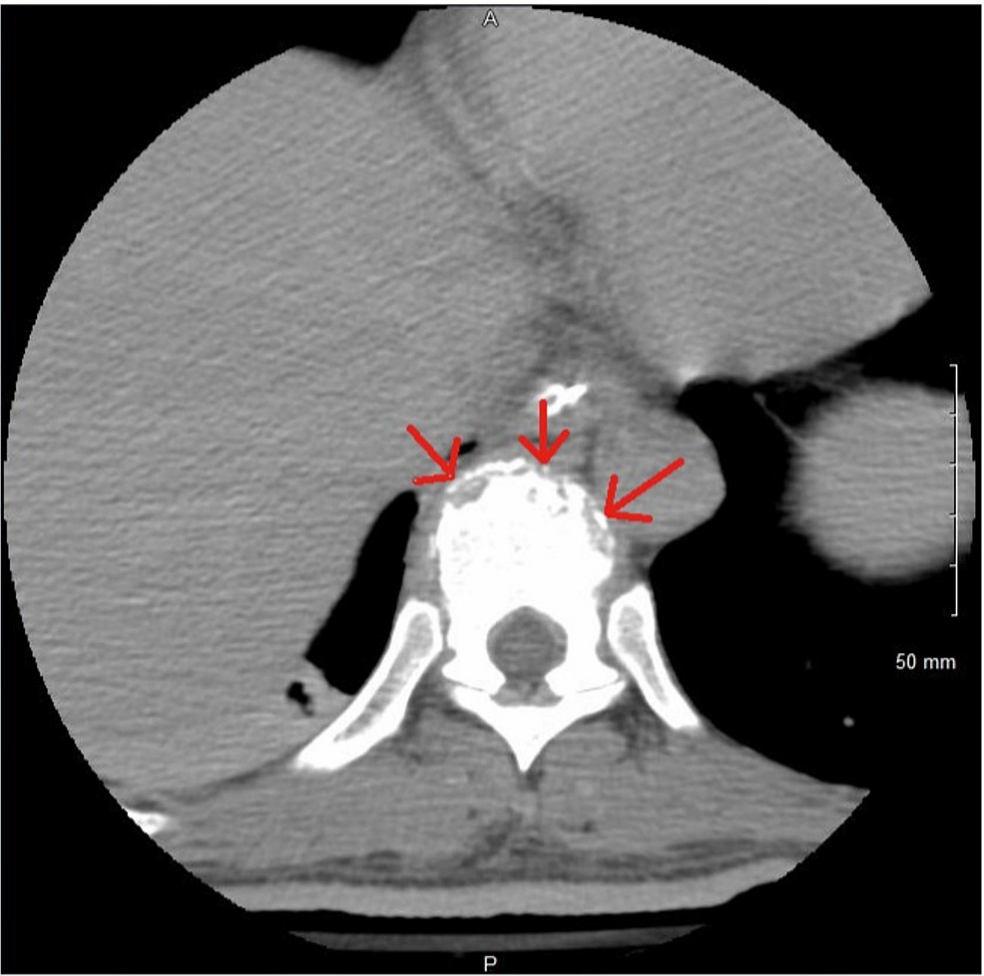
CT spine showing endplate osseous erosions around T10 suggesting vertebral osteomyelitis
